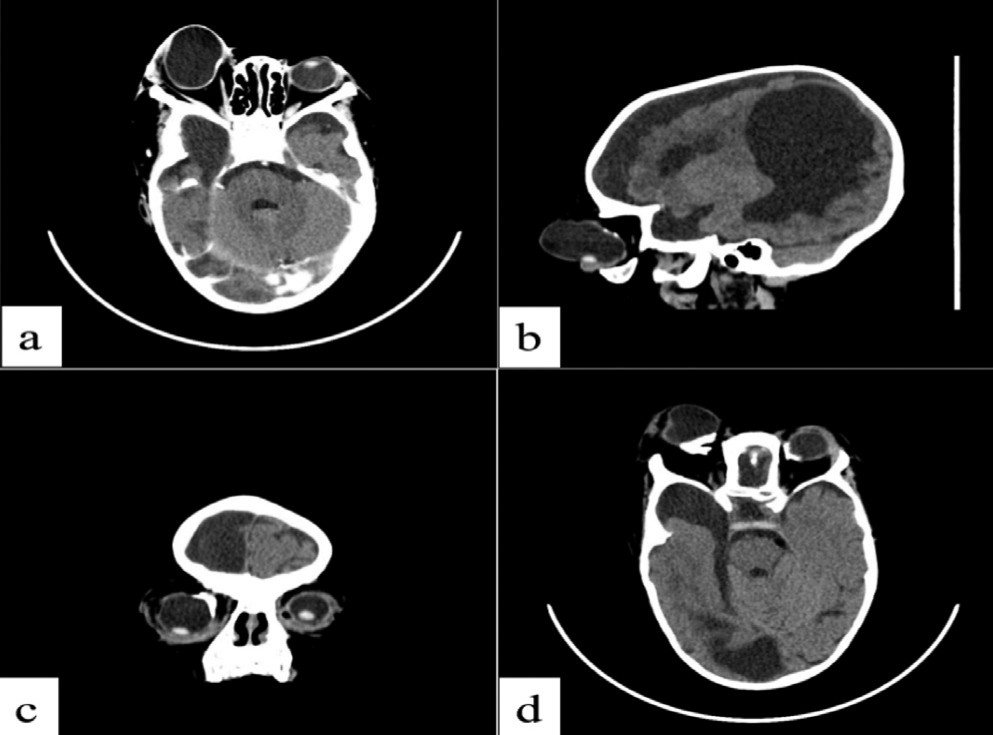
Axial computed tomography of the brain at the level of the orbit showing a cystic right orbital mass on the same side of the cerebral lesion with inferior displacement of the lens of the eye (a-c) and associated calcification of the posterior aspect of the globes of both eyes (d)
